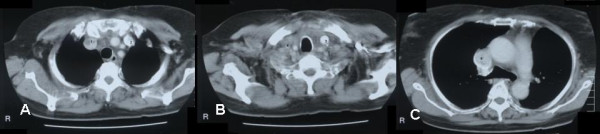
(Case 1).1a CT thorax showing filling defect in the right brachiocephalic vein (1) due to thrombus, while the left brachiocephalic vein (2) is patent and shows intense contrast enhancement. 1b CT neck showing patent left internal jugular vein with intense contrast enhancement due to regurgitation (3). Right internal jugular vein is not seen due to thrombus (4). 1c CT thorax showing blocked superior vena cava (5) with thrombus and a rim of contrast enhancement indicating partial block.
